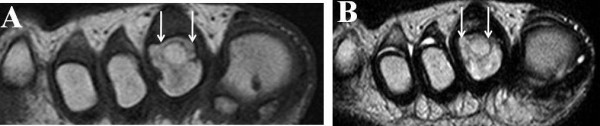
Magnetic resonance imaging (MRI) study of the metatarsophalangeal joint one year after the operation. (A) No low-intensity zone was detected in the second metatarsal head in the axial plane of the T1-weighted MRI scan. (B) No high-intensity zone was detected at the subchondral zone in the axial plane of the T2-weighted MRI study.
